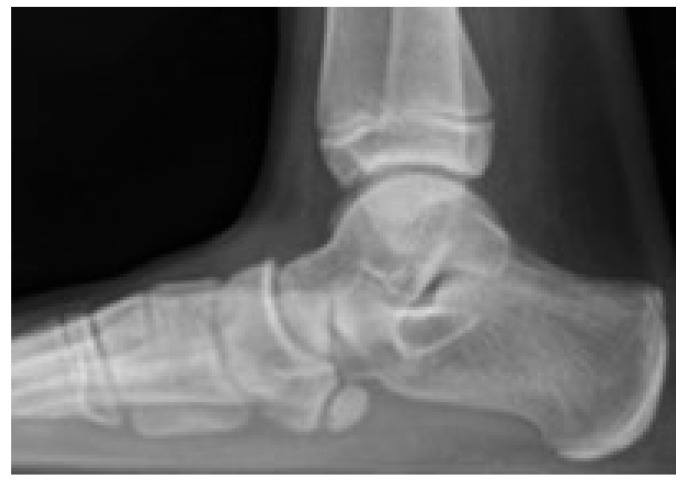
Radiograph (lateral view) of an 11-year-old female patient showing a flatfoot with a large accessory navicular bone.
